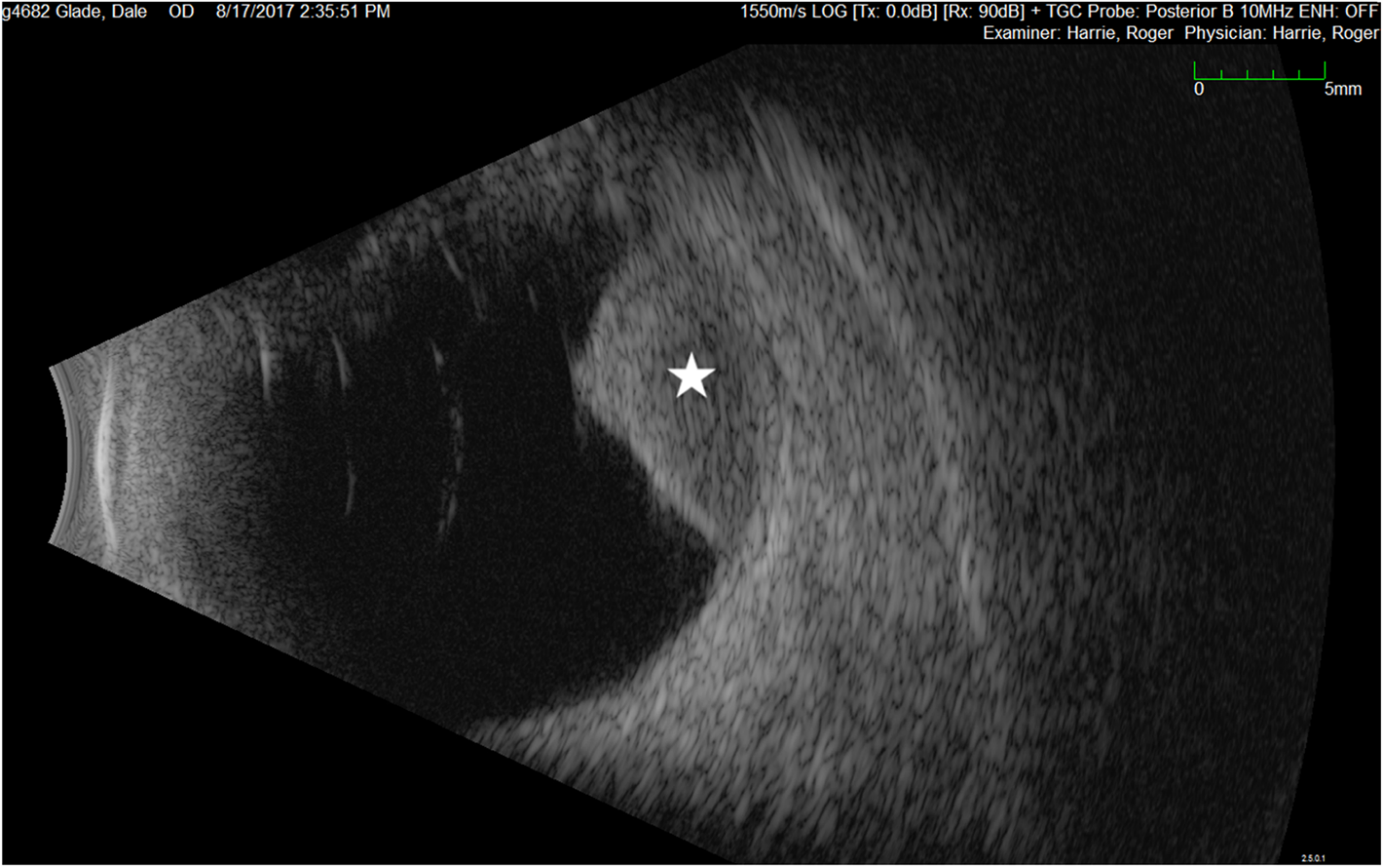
B-Scan Ultrasound of patients right eye showing the classic collar button (mushroom) shape of tumor (white star) without evidence of extrascleral extension
